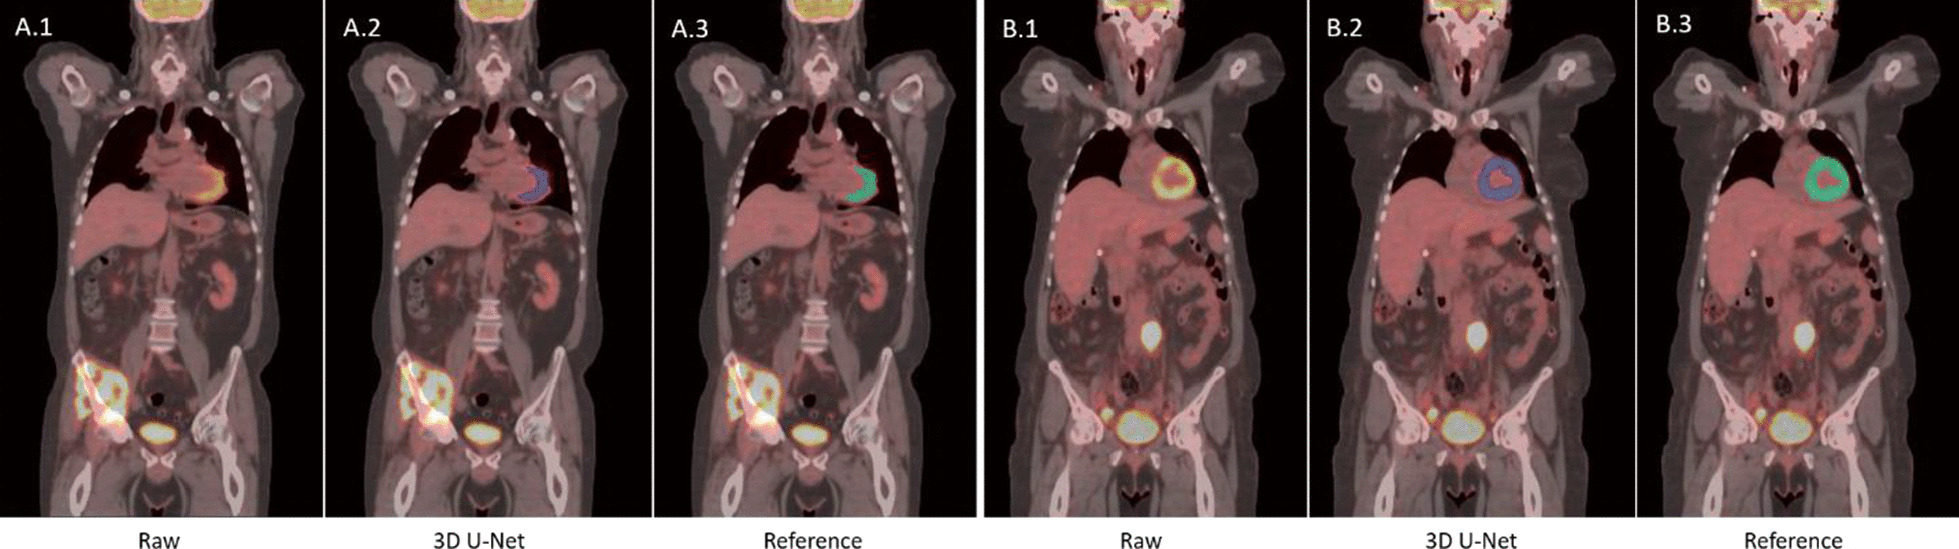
Example of heart segmentation with different degree of FDG uptake in heart: a medium uptake and b high uptake
